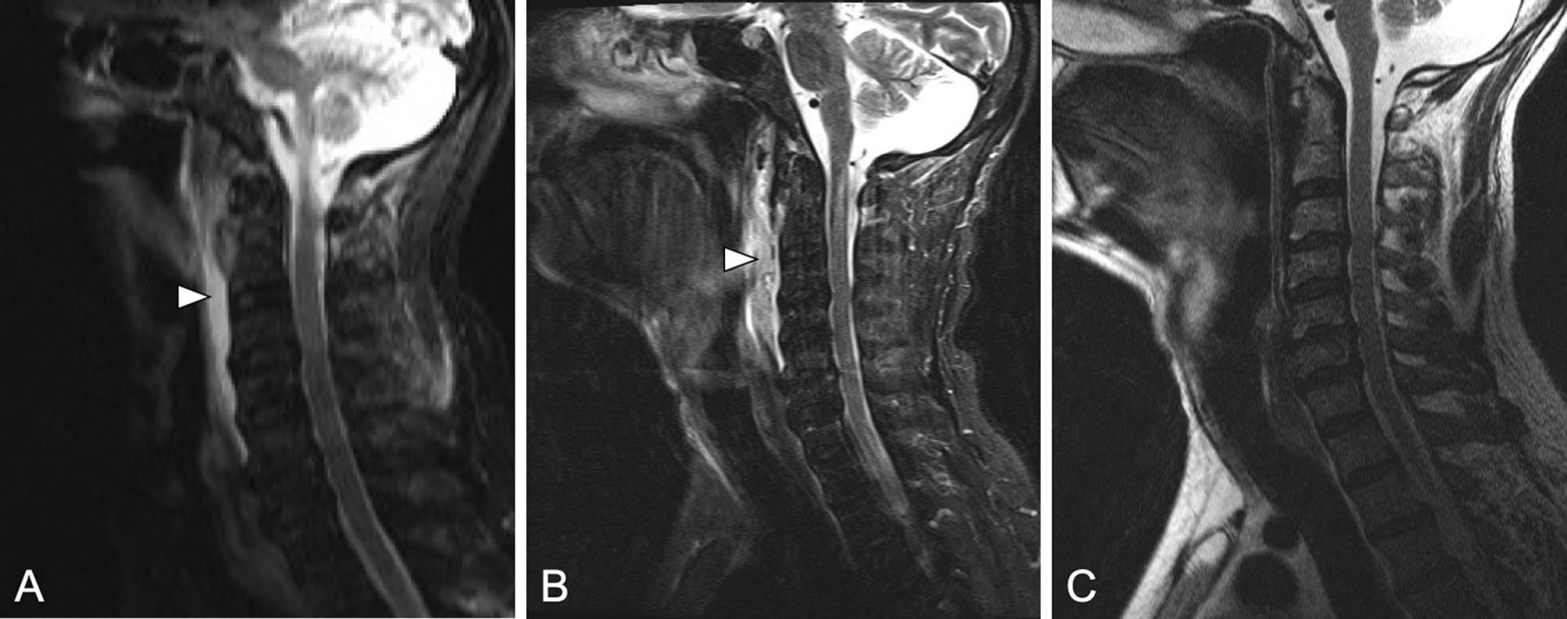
MRI of a 55-year-old male patient presenting with a 2-week history of pain when moving his neck with acute limitation of neck mobility (Patient 2). Upon clinical examination including transnasal fiberoptic endoscopy of the pharynx and larynx, no visible swelling was noted. a Sagittal T2-weighted MR image with fat saturation of the cervical spine showing extensive prevertebral edema at the level of C1–C6 (white arrowhead) and discrete joint effusion of the atlanto-axial joint leading to the diagnosis of RA/septic arthritis. Subsequently, the patient underwent a transcervical exploration of the retropharyngeal space. However, intraoperatively, no abscess formation was found. Six hours after surgery, the patient suffered from a postoperative bleeding from the surgical field that made the revision surgery necessary. As a complication, the patient suffered from a postoperative hypoglossal nerve paresis. b Sagittal T2-weightend MR image with fat saturation 5 days postoperatively showing increased effusion with postoperative edema (white arrowhead). At this time, neck pain had decreased under antibiotic and analgetic treatment. c Sagittal T2-weightend MR image with fat saturation 6 weeks postoperatively showing complete resolution of MRI findings
